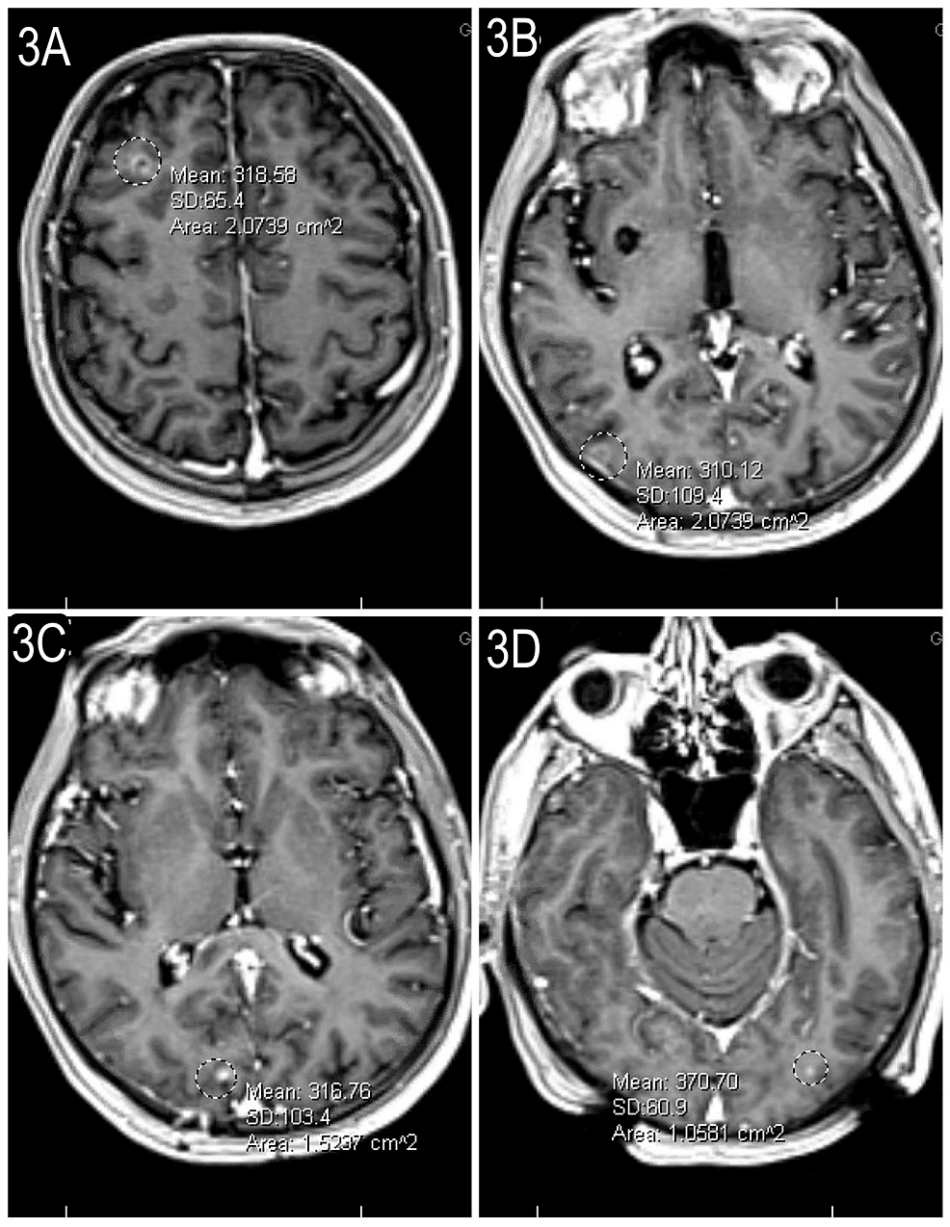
Newly Identified Brain Masses Discovered Following Eight Months of Treatment Signifying Disease Progression 3A-3D: These images were collected from the brain MRI the patient received following eight months of osimertinib to monitor the progression of the original brain metastasis in addition to checking for potential new masses. These images show different new brain metastases that were discovered throughout the brain eight months after initial diagnosis and discovery of spread to the brain. Following eight months of treatment, multiple new masses were identified, which are visualized in this figure, signifying the progression and further spread of the disease despite treatment.
